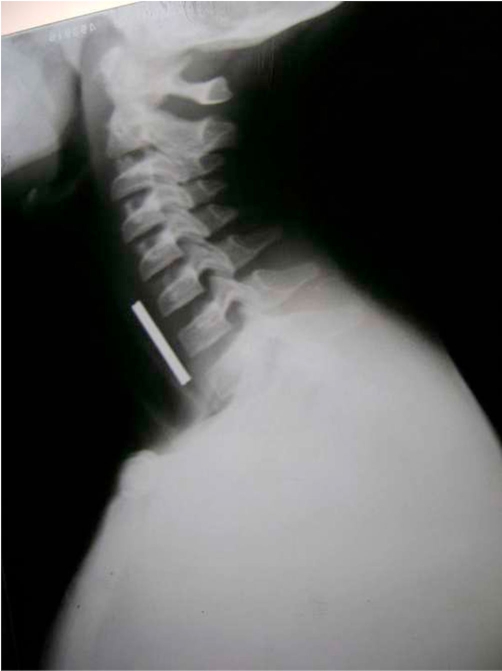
A.P. View X-ray chest showing coin in upper esophagus.
